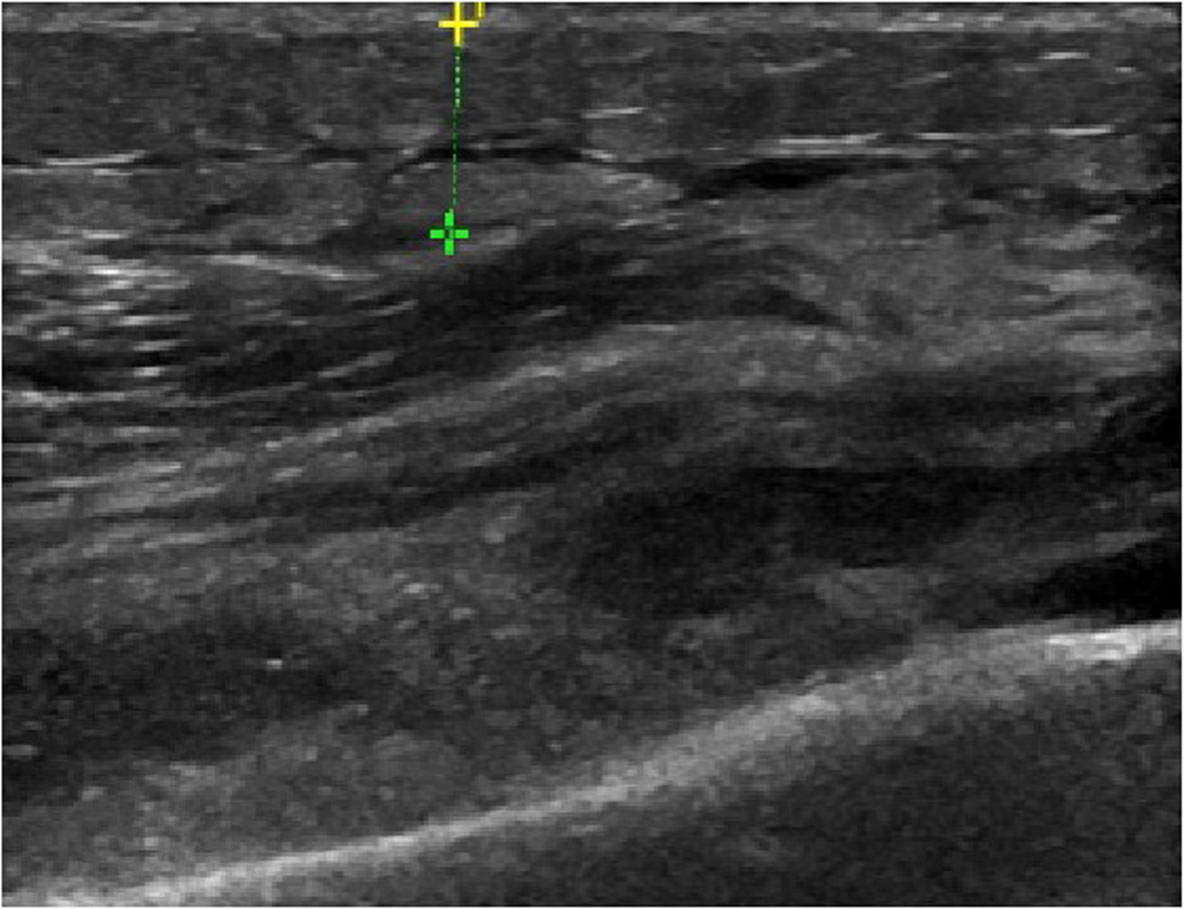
Ultrasound scan on the left knee joint which was suspected to AL case after TKA
